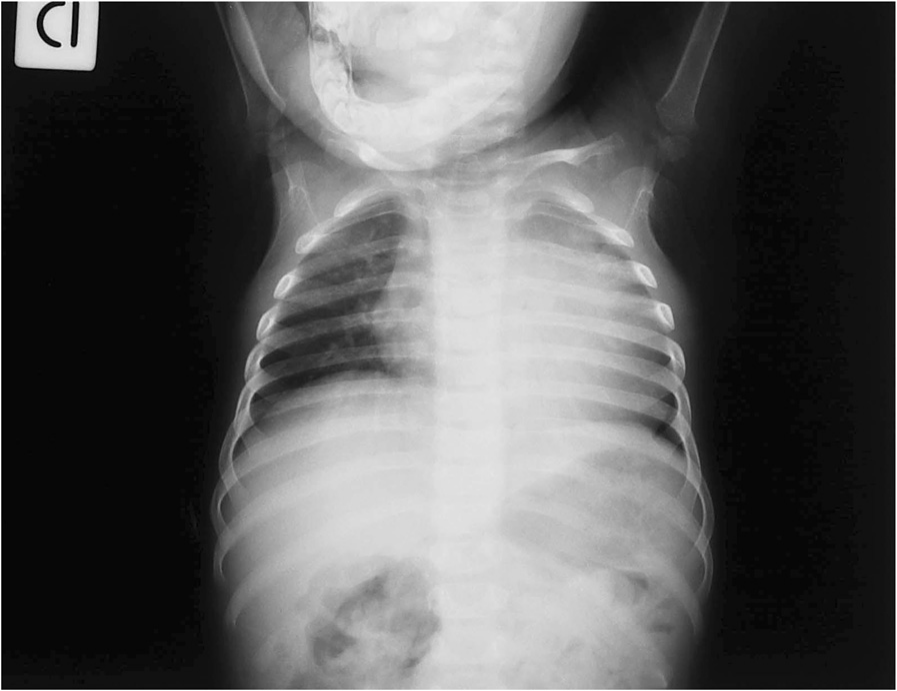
Chest x-ray showing widespread lung hyperinflation and severe cardiomegaly.
